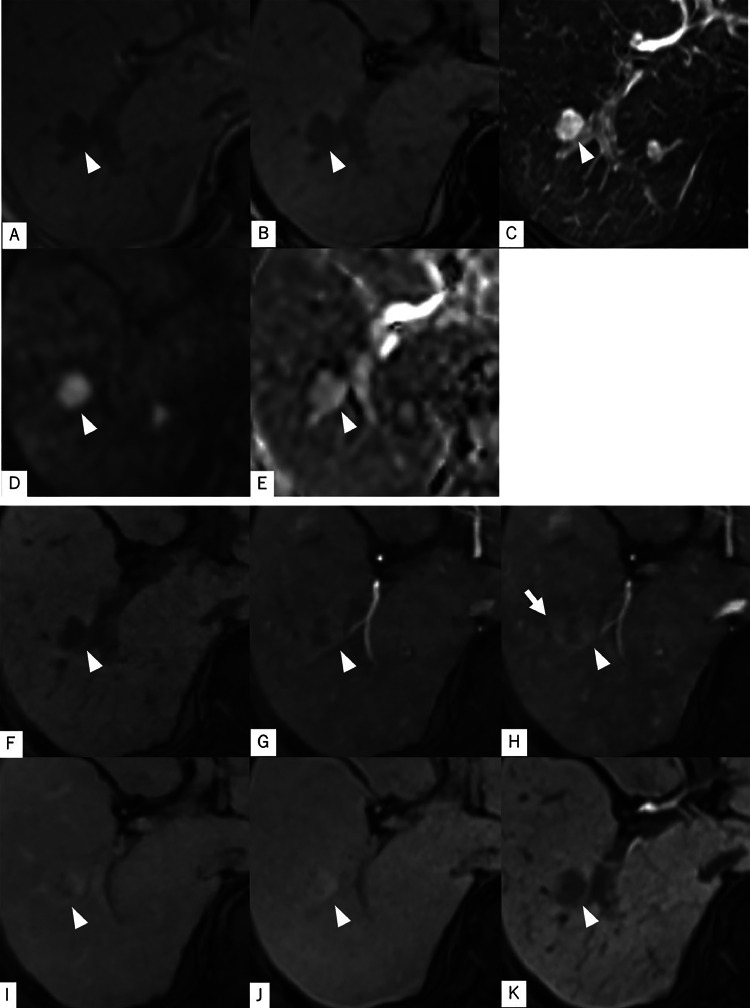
There was no signal reduction in the comparison of the in-phase (A) and opposed-phase (B) images of T1WI. In T2WI (C), a clear high signal was observed, consistent with the high signal intensity typically seen in solid mass lesions, such as HCC. In DWI (D), there was a high signal, but it also showed a high signal on the ADC map (E), which was considered T2 shine through, rather than diffusion restriction. On dynamic contrast enhancement, we observed a gradual contrast effect spreading from the left side of the lesion to the entire lesion from the early arterial phase (G) to the transition phase (J). In the hepatobiliary phase (K), it showed a lower signal than the surrounding liver. In the late arterial phase (H), a localized enhancement around the lesion, which did not show any signal change on plane MRI, was observed, raising suspicion of an AP shunt or drainage blood flow from the tumor (corona enhancement).
